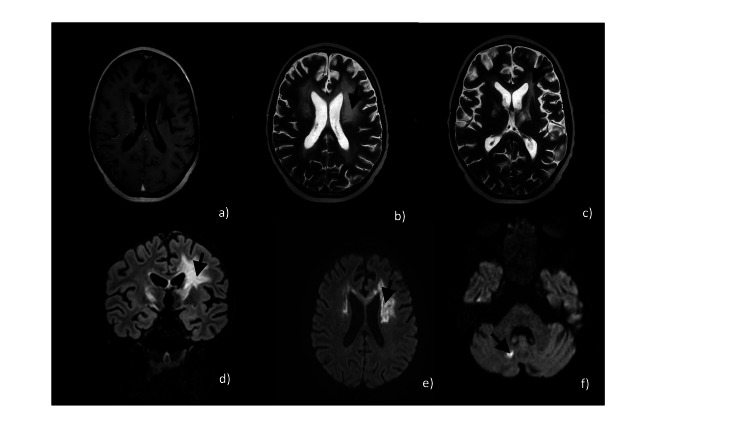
Hypointense area in T1 axial image post-contrast (a); hyperintense area in T2 ((b) and (c)) and T2-FLAIR (d) axial images without diffusion restriction ((e) and (f)); and without peripheral contrast enhancement (a), located in the cerebral and cerebellar white matter. FLAIR: Fluid-attenuated inversion recovery
